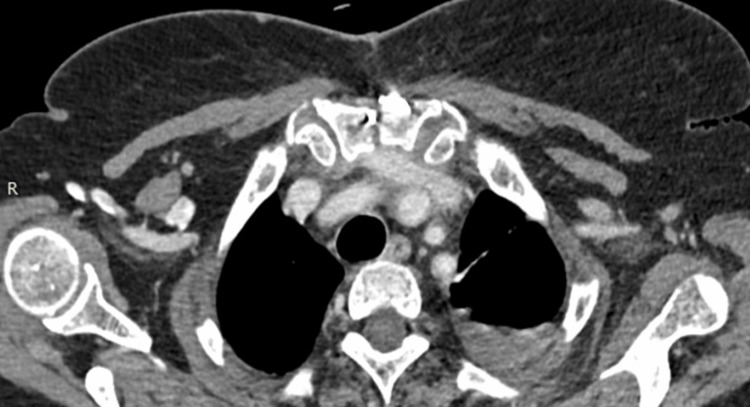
Postoperative computed axial tomography: The control CT scan with contrast material shows that the prosthetic brachiocephalic vein is patent and both lungs are fully dilated.
